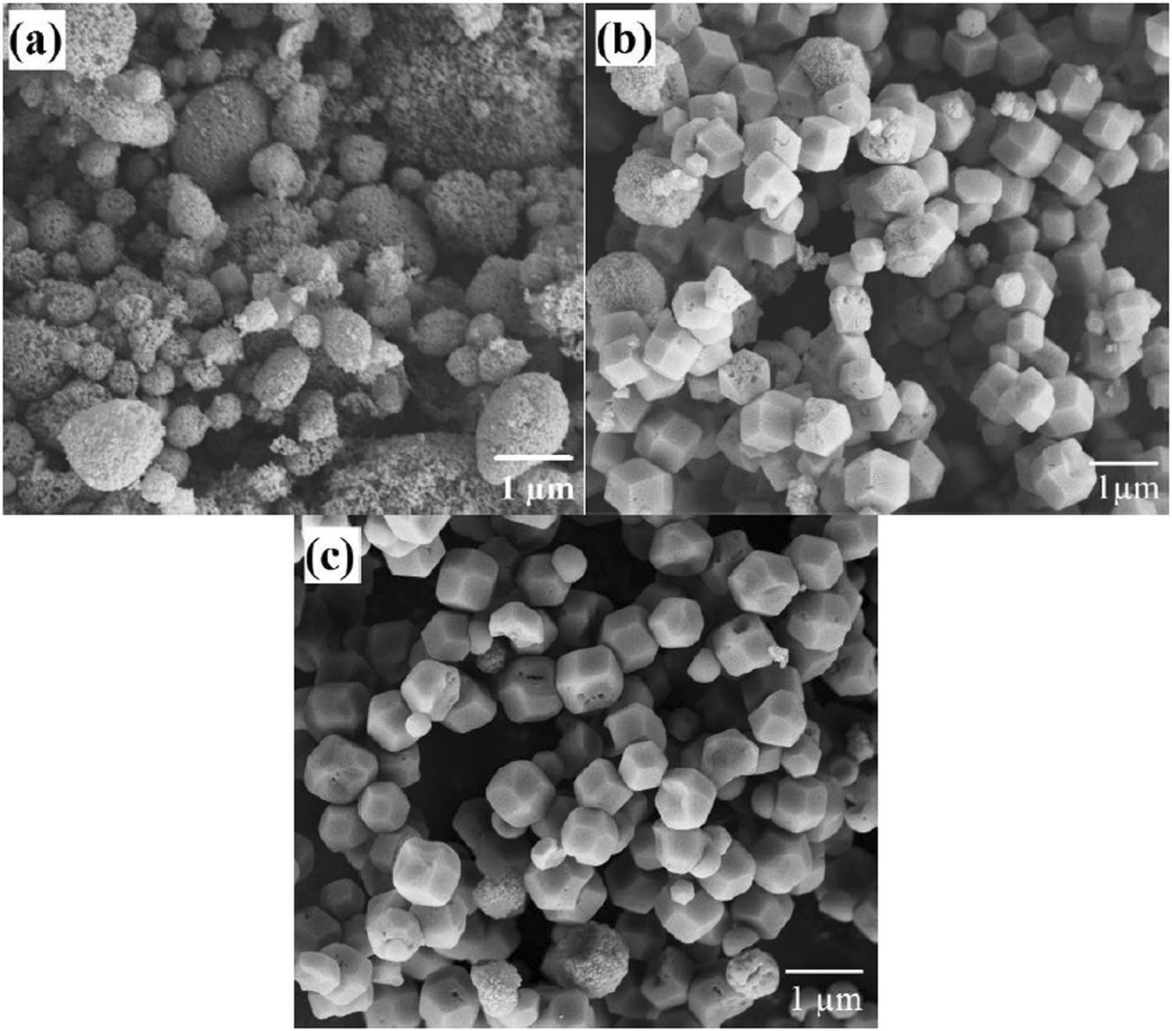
FESEM images of the samples pretreated with 60 minutes of ultrasonic irradiation and followed by hydrothermal heating duration (a) 0.5 days (S2), (b) 1 day (S3) and (c) 2 days (S5).
